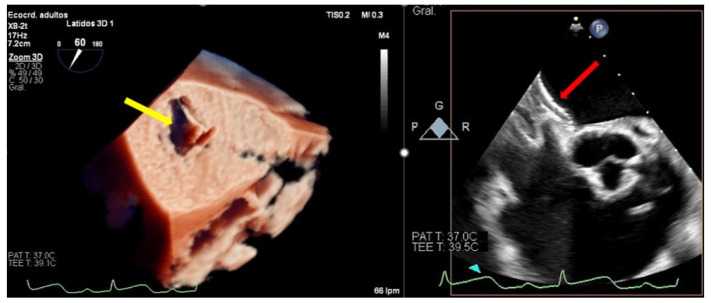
Persistent atrial septal defect. (Left) Three-dimensional transesophageal echocardiogram image showing a large atrial septal defect with tearing of the tissue through the transseptal puncture area (yellow arrow) from the left atrium. (Right): Two-dimensional transesophageal echocardiogram showing an Amplatzer device closing the iatrogenic atrial septal defect (red arrow).
